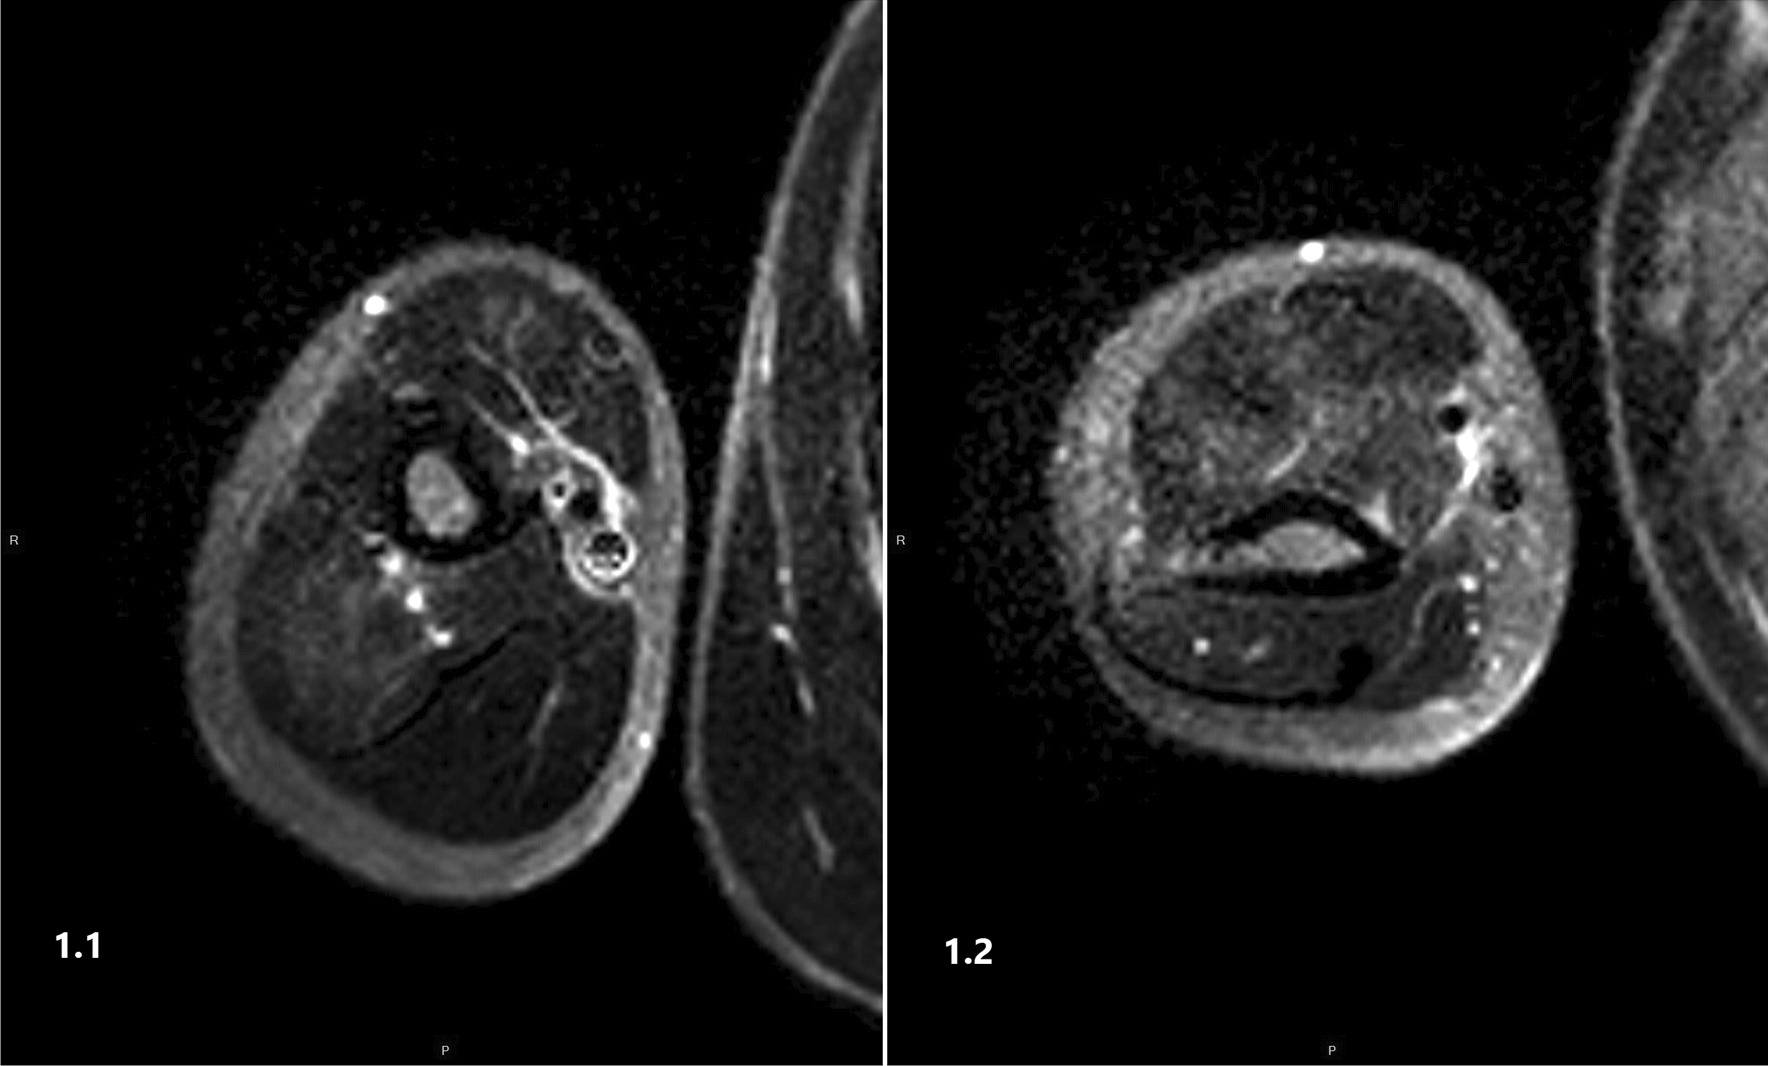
MRI of the right arm, (Images 1.1, 1.2) of patient number 1, demonstrates a high T2FS signal (along Triceps, Biceps and Brachialis) compatible with myositis.
